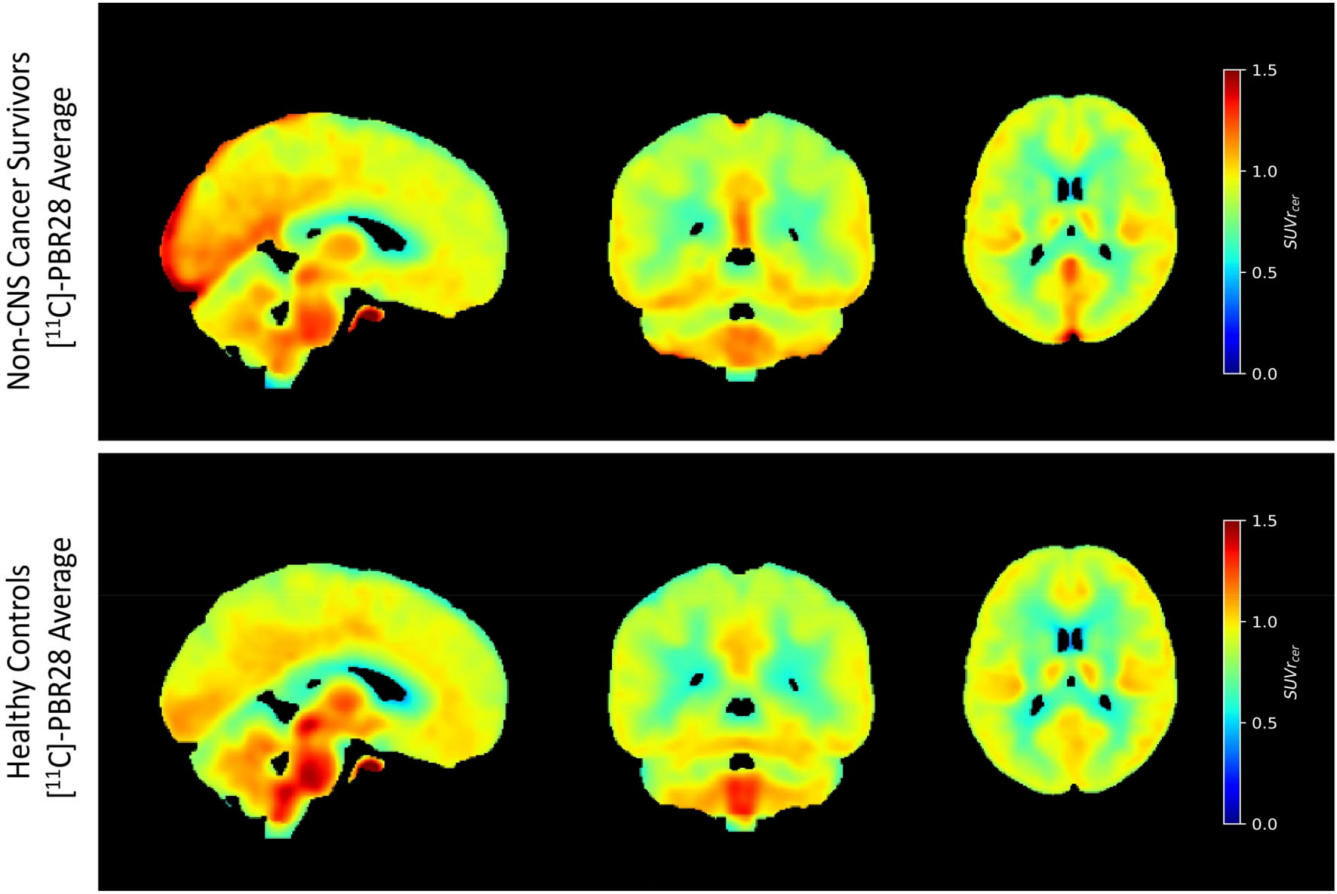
Average [^11^C]‐PBR28 standardized uptake value ratio (SUVR) maps registered to AssemblyNet standard space for survivors of head and neck cancers (*n* = 10) versus matched healthy controls (*n* = 10) are displayed in orthogonal views. The whole cerebellum was used as a reference region for SUVR calculation according to previous studies and standardized protocols. Cancer survivors have greater uptake of TSPO tracer [^11^C]‐PBR28 in posterior and medial regions compared to matched healthy controls.
